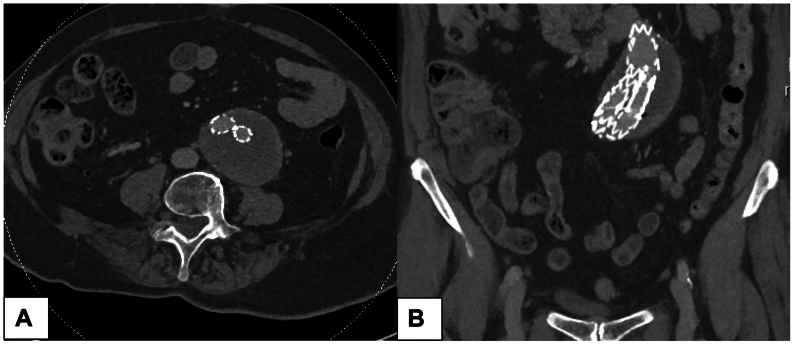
Initial computed tomography (CT) angiogram showing axial (A) and coronal (B) views on first follow-up CT scan after endovascular aneurysm repair (EVAR).
